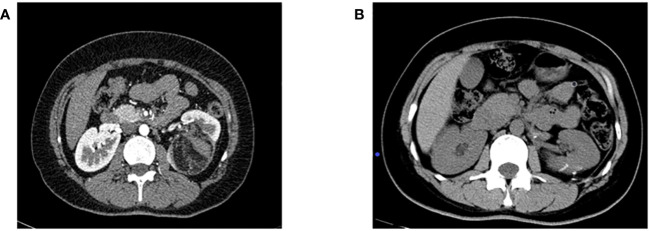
(A) Preoperative enhanced CT imaging results; (B) CT results of the patient 6 months after surgery.
